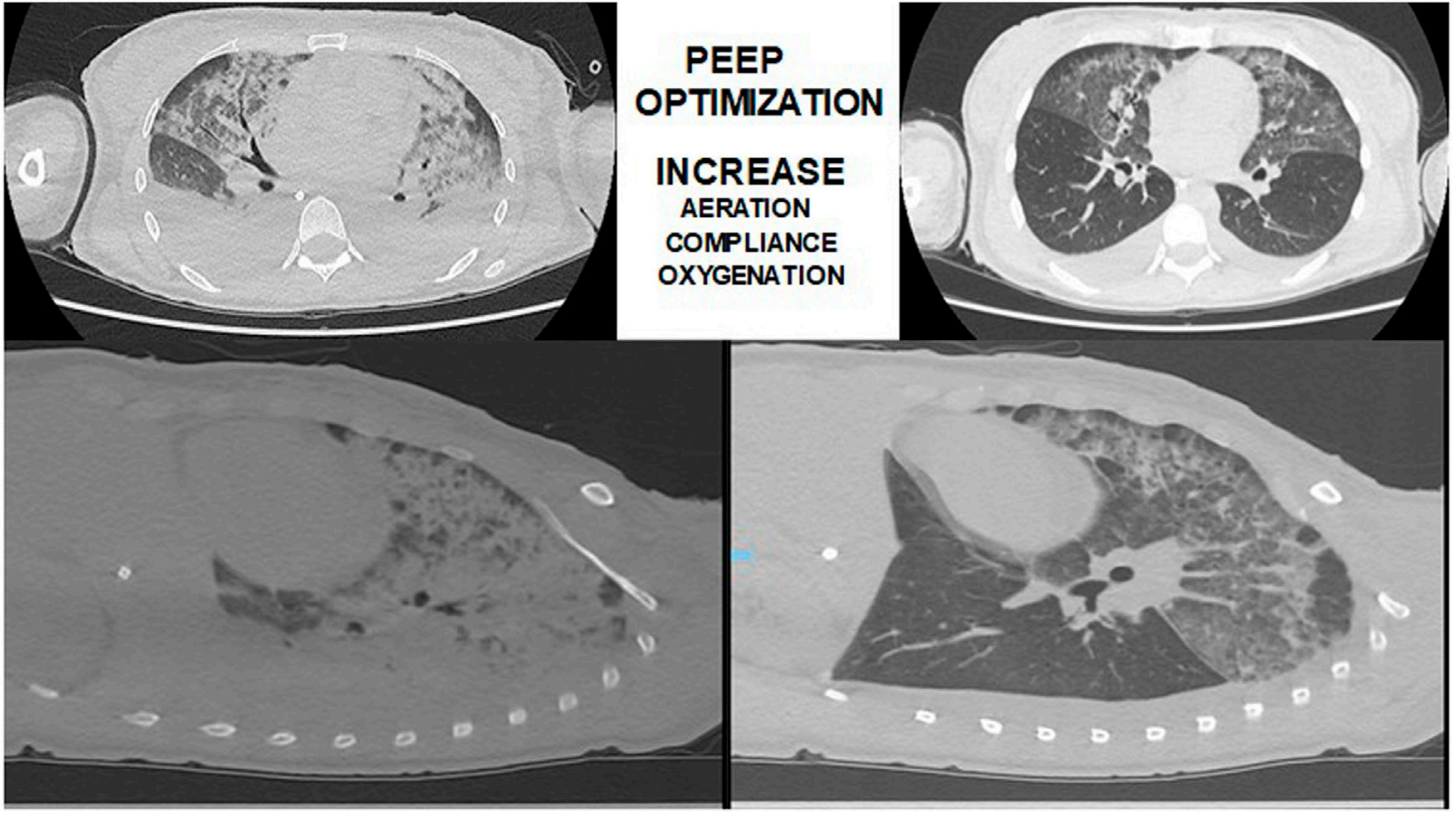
Thoracic-computed tomography guided recruitment and PEEP titration in a patient with ARDS (authors own material from reference 14).
